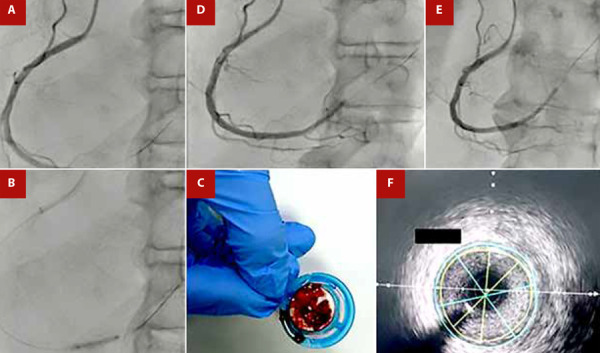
A) Initial Coronary Angiography showing thrombotic occlusion in the right coronary artery. B) Balloon angioplasty with a semi-compliant balloon of 2.0 x 15 mm up to 14 atm. C) Mechanical thrombus aspiration obtaining abundant thrombus material. D) and E) Post-mechanical thrombus aspiration with improvement of distal flow. F) Intravascular ultrasound (IVUS) in the right coronary artery performed at 48 hours showing no evidence of atherosclerotic lesion, thrombus, or dissection.
